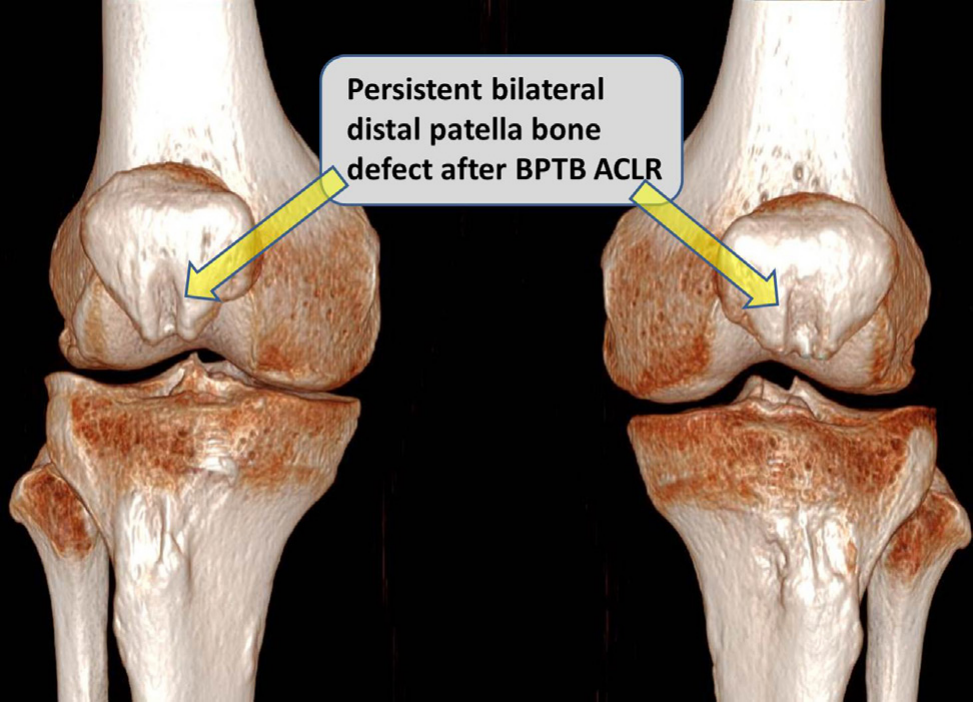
Three dimensional computed tomography images of right and left knees showing persistent patellar bone defects after bilateral autologous bone–patellar tendon–bone primary anterior cruciate ligament reconstructions.2.Tunnel aperture locations are assessed. In this case, both seem appropriately located (Fig 2).
